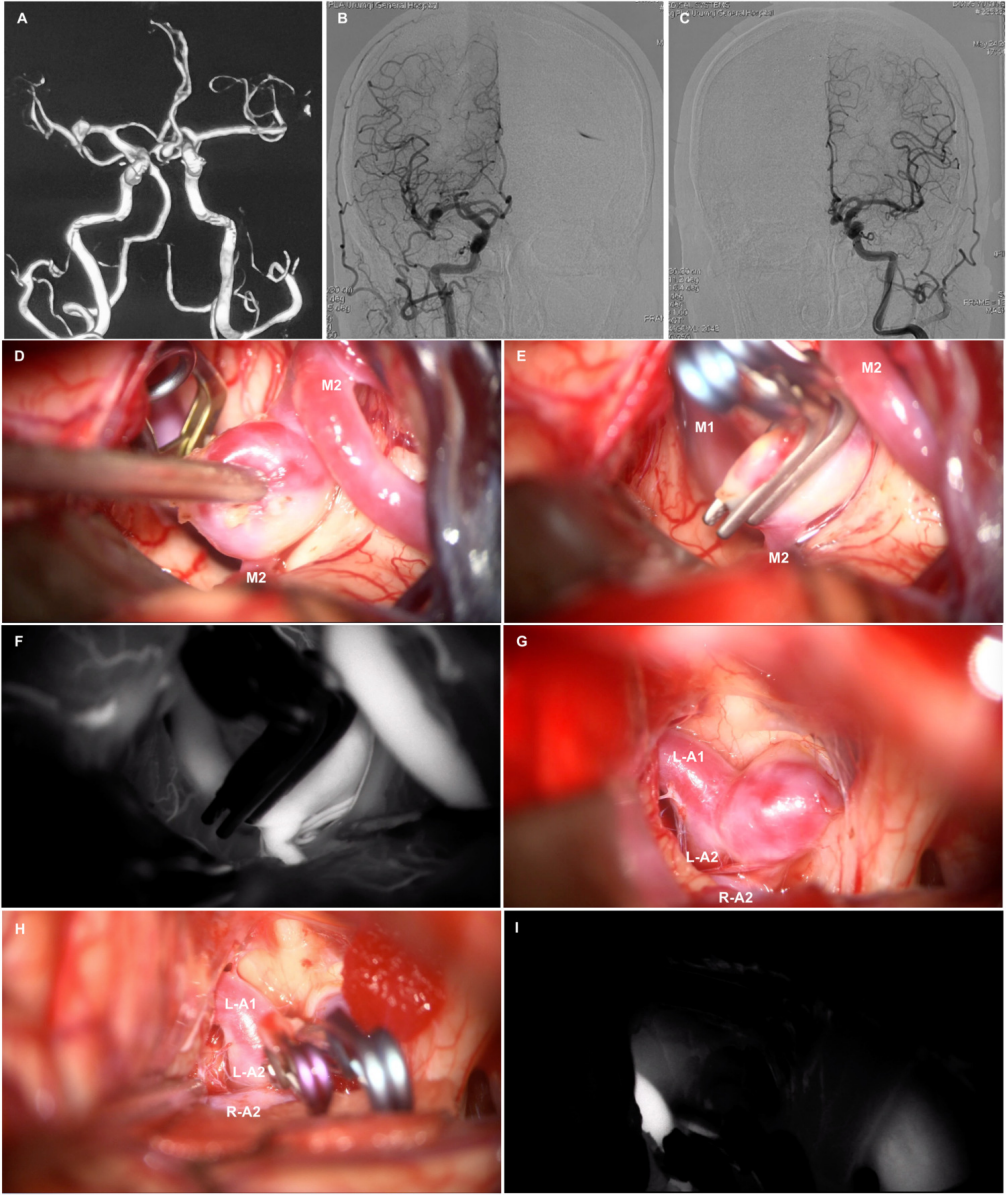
Brain CTA (A) reveals multiple intracranial aneurysms in the ACoA. and right MCA bifurcation. Anteroposterior view of the right (B) and left (C) internal cerebral artery (ICA) angiogram. Intraoperative photos show perpendicular clipping in the right MCA bifurcation aneurysm (D,E), and multiple clipping in the ACoA aneurysm (G,H). Intraoperative indocyanine green angiography confirmed complete obliteration of the aneurysm and patency of the parent vessels (F,I).
